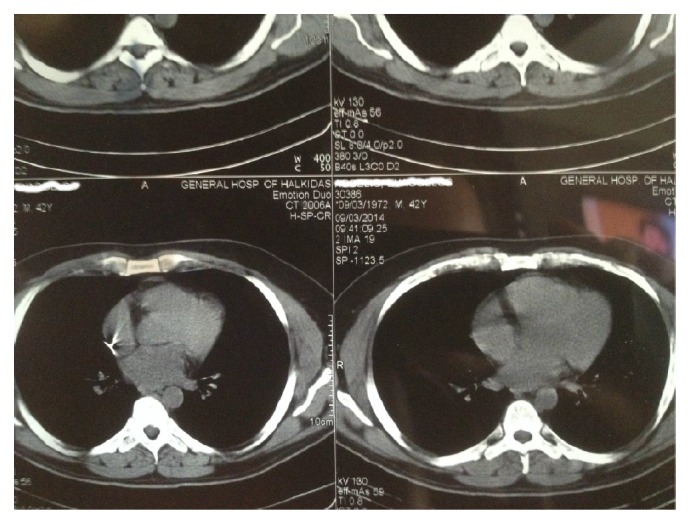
Computed tomography (CT) of the thorax. A small-sized metallic artifact lies at the opening of the right atrium of the heart. No other cardiac or mediastinal injury.
